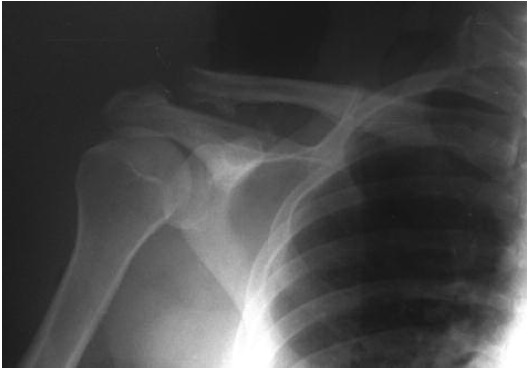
Radiograph showing bony spur followingdistal clavicle
